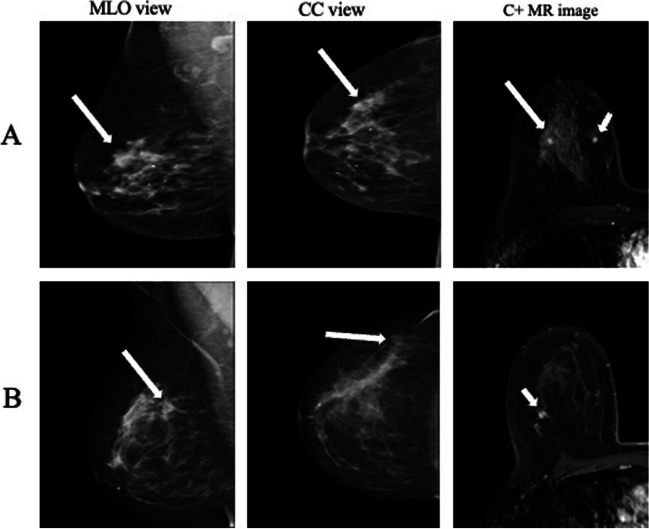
Incidental MRI-detected lesions not visible on bilateral mammograms or ultrasound were biopsied if they were BI-RADS 3–5 lesions. Two cases of incidental lesions (a fibroadenoma of 5 mm (A) and a carcinoma of no special type of 7 mm (B)) detected in women in their mid-60s with breast cancer were not detected prior to MRI on either mammography or ultrasound. Long arrows point to the primary tumor and short arrows to the incidental MRI-detected lesion. MLO, mediolateral oblique; CC, craniocaudal; C+, contrast enhanced
